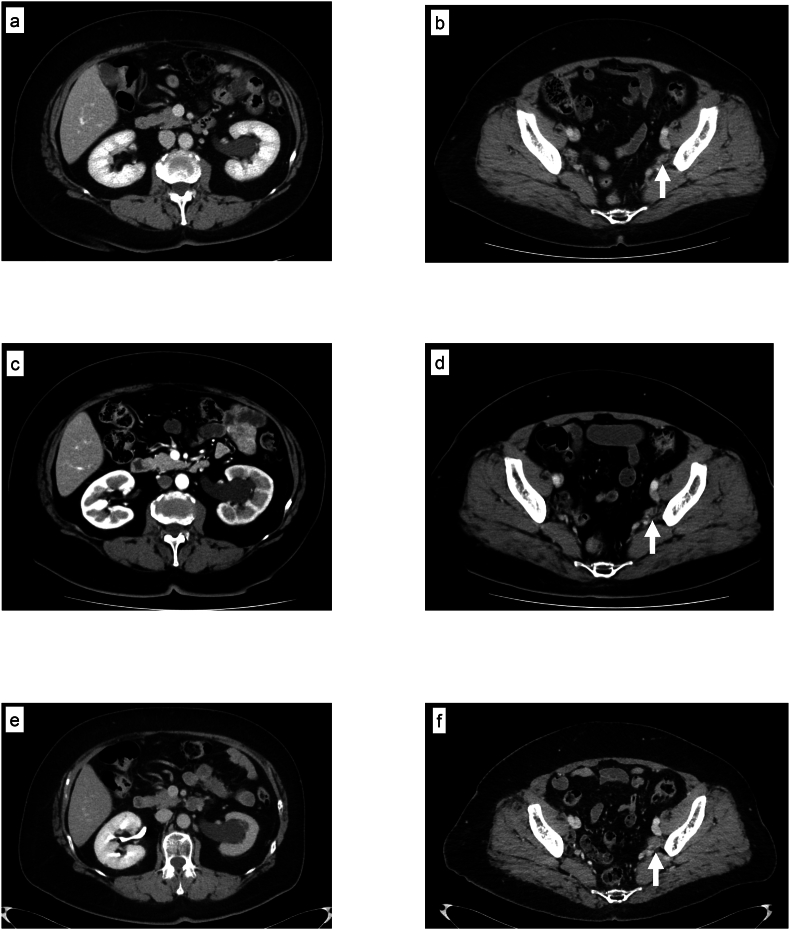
CT images during follow-up. (a, b) Left hydronephrosis (a) and contrast-enhanced lesion in the left distal ureter (b, white arrow) at the time of hydronephrosis detection. (c, d) Slightly decreased enhancement of the left kidney with hydronephrosis (c) and enhanced lesion of same size in the left ureter (d, white arrow) at two months after detection. (e, f) Atrophy and diminished enhancement of the left kidney (e) and an increased size of enhanced lesion (34 × 20 × 20 mm^3^) in the left ureter (f, white arrow) at five months after detection.
